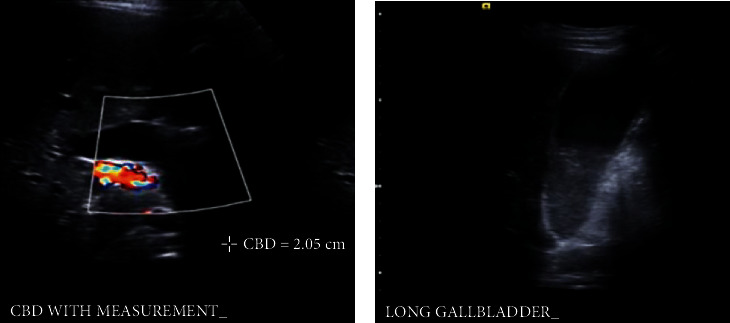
Abdominal ultrasound revealing dilated common bile duct measuring (a) 2.05 cm and gall bladder with (b) biliary sludge.
